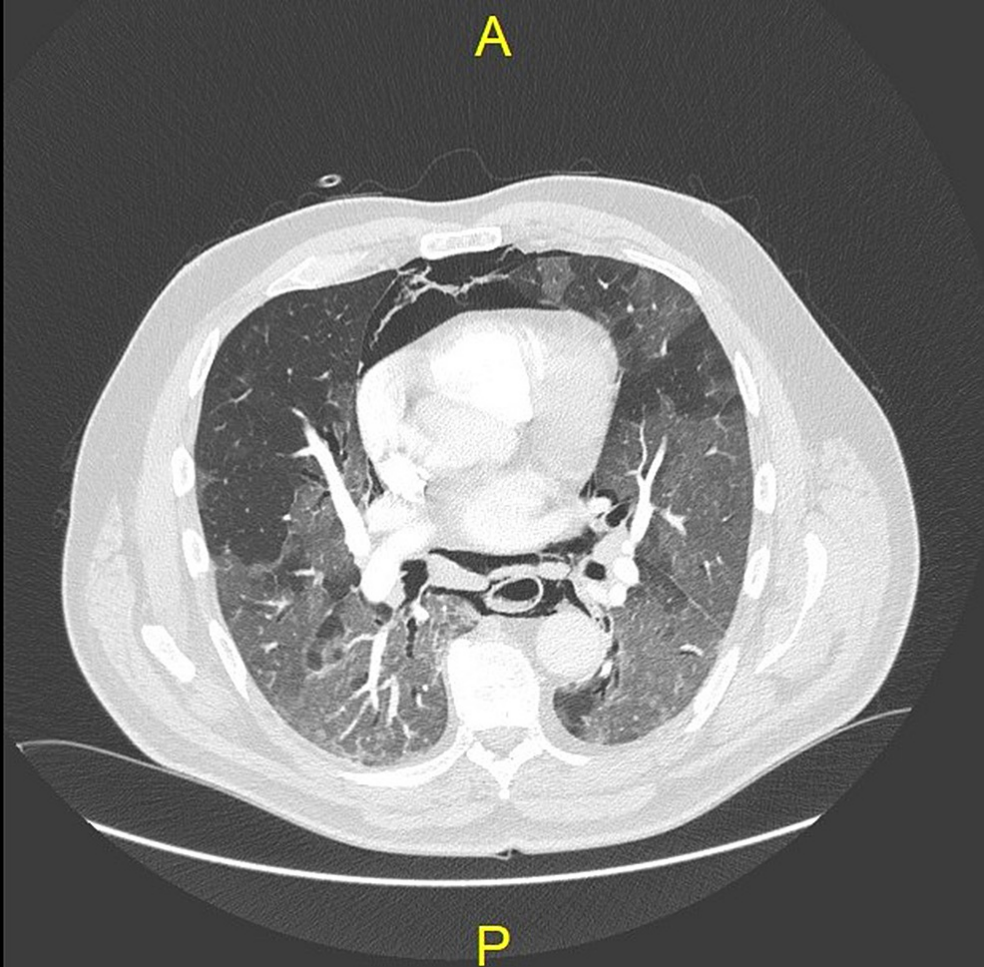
Coronal CTPA slice demonstrating extensive pneumomediastinum and associated COVID-19 pneumonitis CTPA: computed tomography pulmonary angiogram; COVID-19: coronavirus disease 2019
